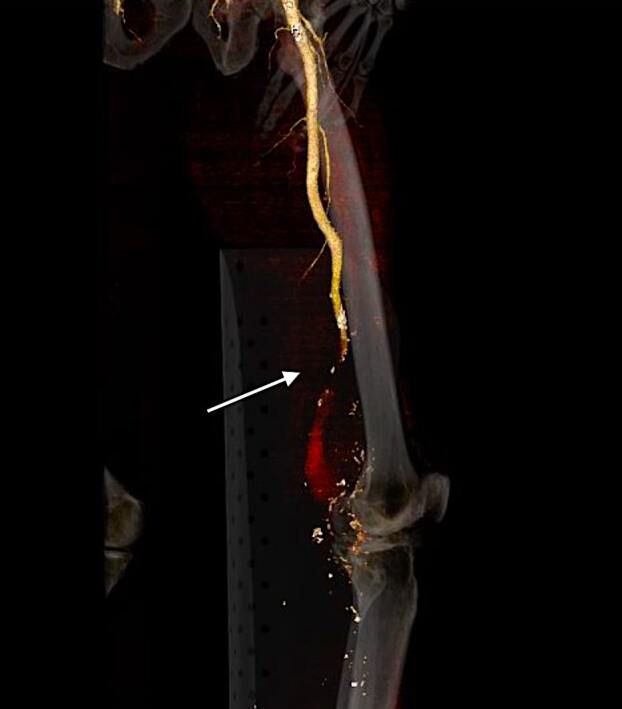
Computed tomography angiography volume-rendering technique reconstruction of the left lower extremity in sagittal view demonstrating superficial artery occlusion with popliteal artery aneurysm rupture (arrow) and lack of arterial contrast enhancement distally.
